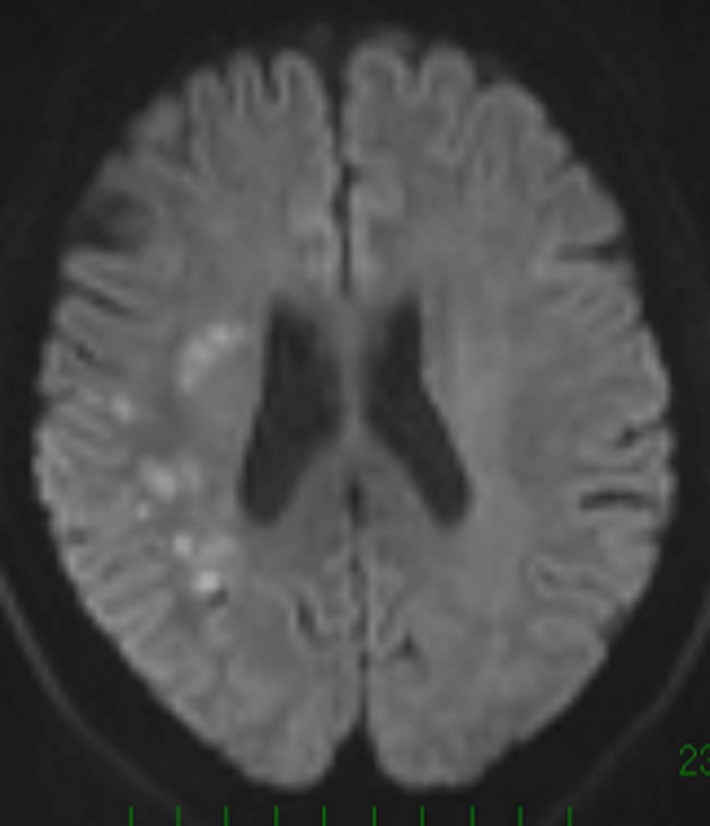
MR imaging (diffusion weighted imaging sequences) revealed multifocal hyperintense signal in a distribution pattern compatible with watershed infarcts in the right posterior MCA territory
